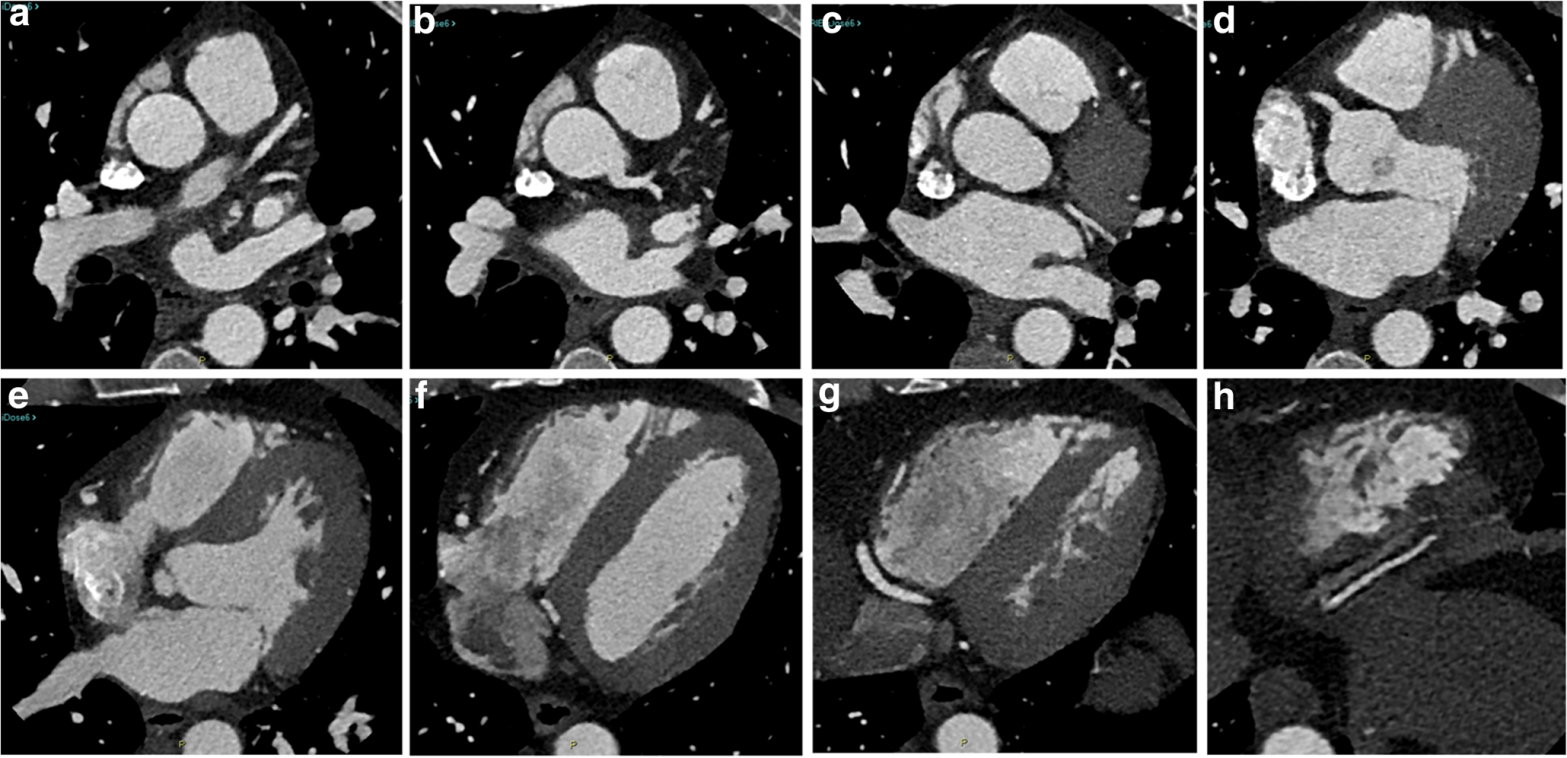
An example of axial TRO-CT images (a–h) used for coronary arteries post-processing
